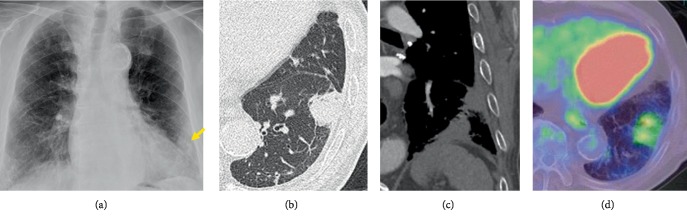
(a) Chest X-ray revealing an irregular shadow in left lower field (arrow). (b) Chest computed tomography (CT) in the lung window revealing a tumor located at S8 of the left lower lobe. (c) Chest CT in the mediastinal window showing a tumor with suspected pleural invasion. (d) Fluoro-2-deoxyglucose-positron emission tomography/CT showing a maximum standardized uptake value of 3.8 in the tumor.
